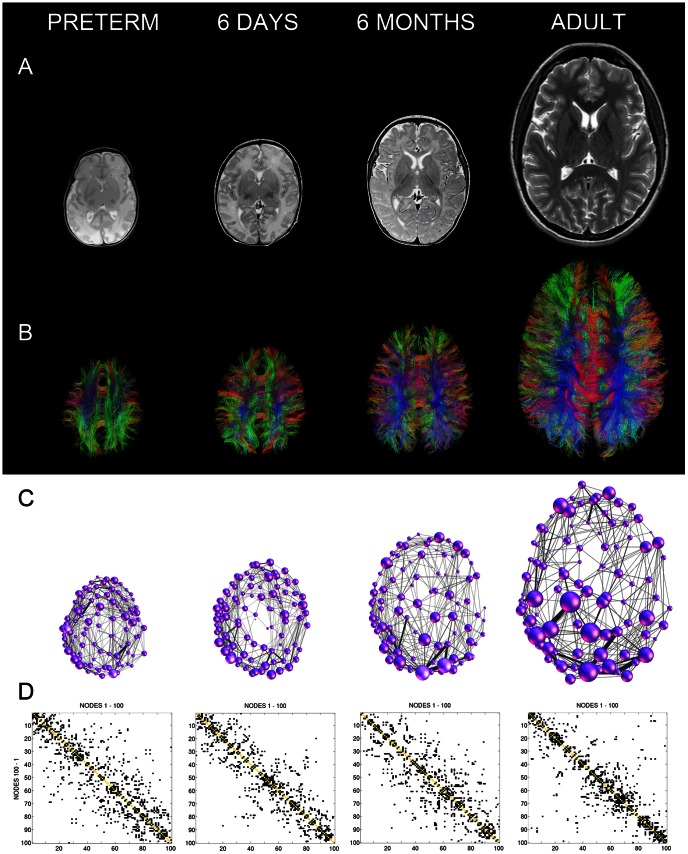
Maturation of the “baby connectome”: examples of brain networks at four different ages. (A) Anatomic MRI images (3T, T_2_-weighted fast spin-echo pulse sequence, echo train length 16, TR/TE = 5000/120 ms, 512×512 matrix, in-plane resolution 0.4×0.4 mm^2^, slice thickness 3 mm, 2 averages). (B) Tractograms reconstructed based on DTI data. Visualization: minimum length 15 mm, skip 90%. (C) Brain networks represented as weighted graphs. The size of the nodes is proportional to the node degree. The edge weights are proportional to the streamline count. (D) Binary connectivity matrices, reordered in a way that maximizes the number of connections close to the main diagonal (1,000,000 reordering attempts). *Note: the 6 days and 6 months networks were mapped in the same infant longitudinally.*
